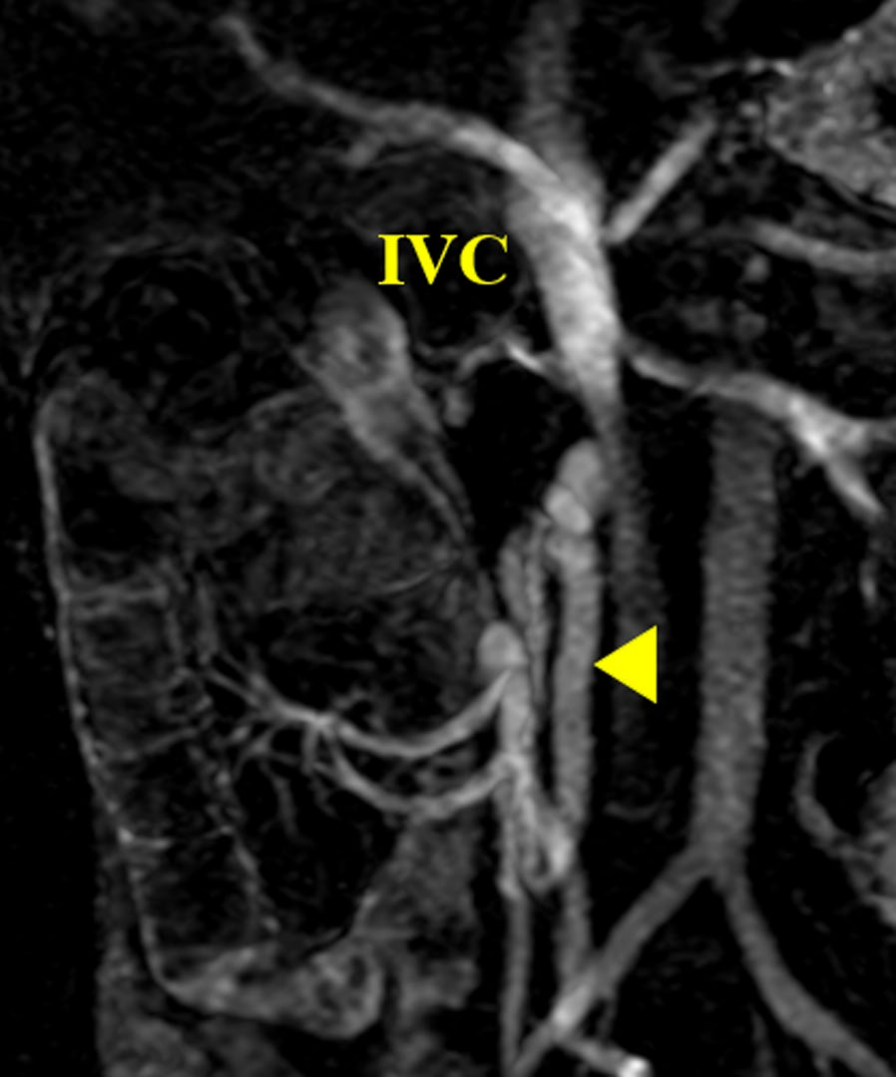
Postoperative findings of contrast-enhanced magnetic resonance imaging (coronal image). Blood flow in the right testicular vein is maintained (arrowhead) and the shunt is open
